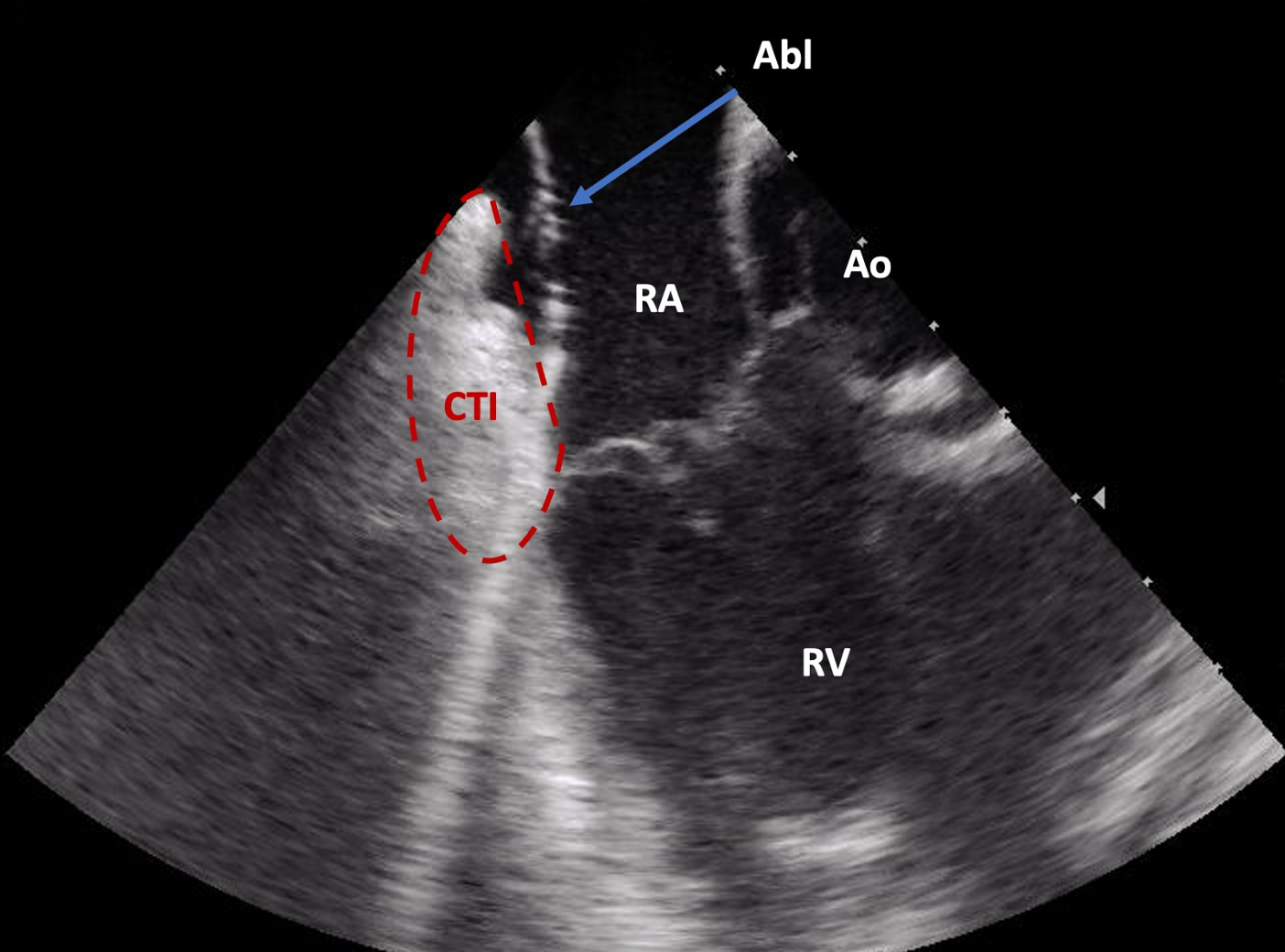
Direct visualization of the ablation catheter on the cavotricuspid isthmus by intracardiac echocardiography (ICE). Abl, ablation catheter; Ao, aortic root; CTI, cavotricuspid isthmus; RA, right atrium; RV, right ventricle.
